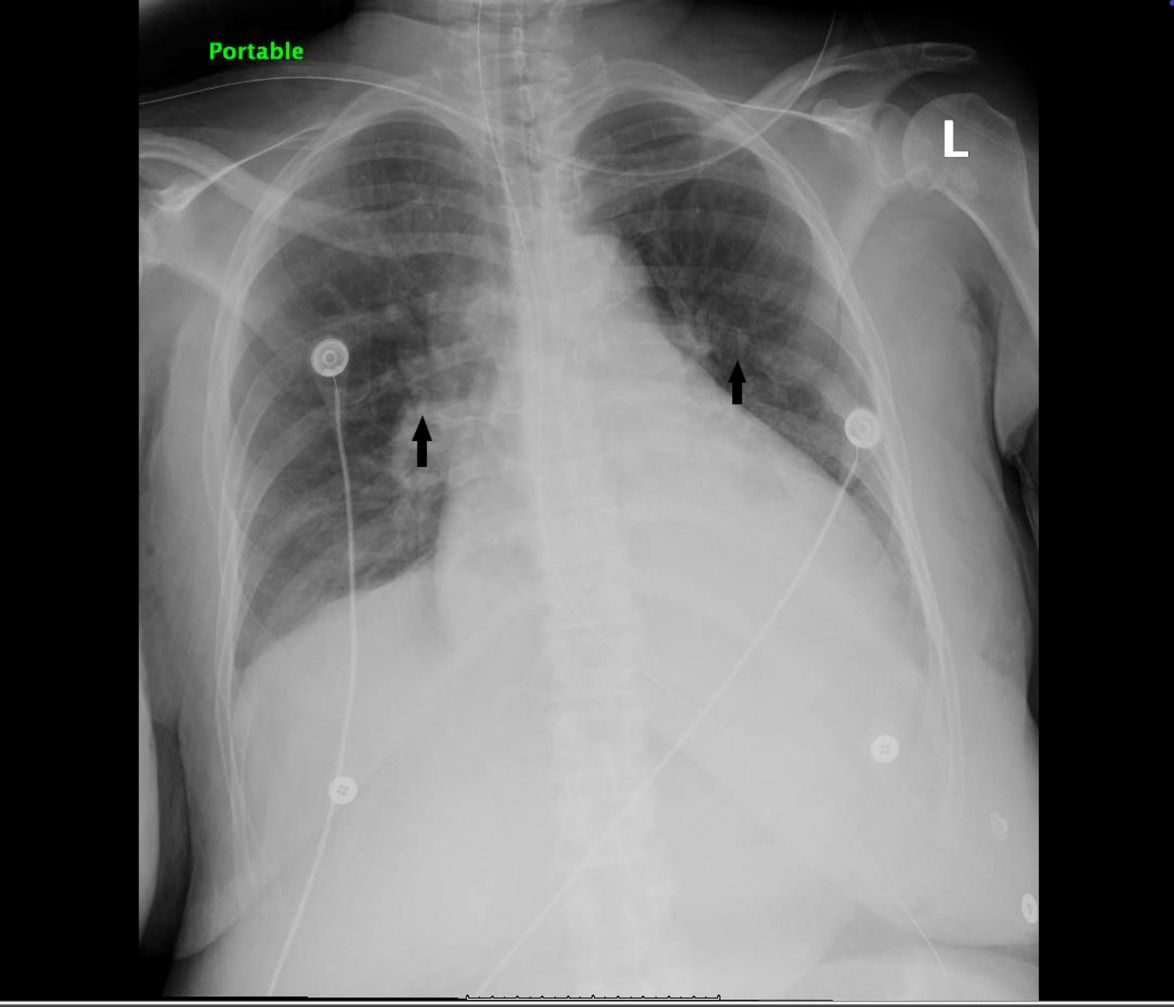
Chest X-ray showing infiltrates in bilateral lungs Infiltrates are indicated by the arrows
